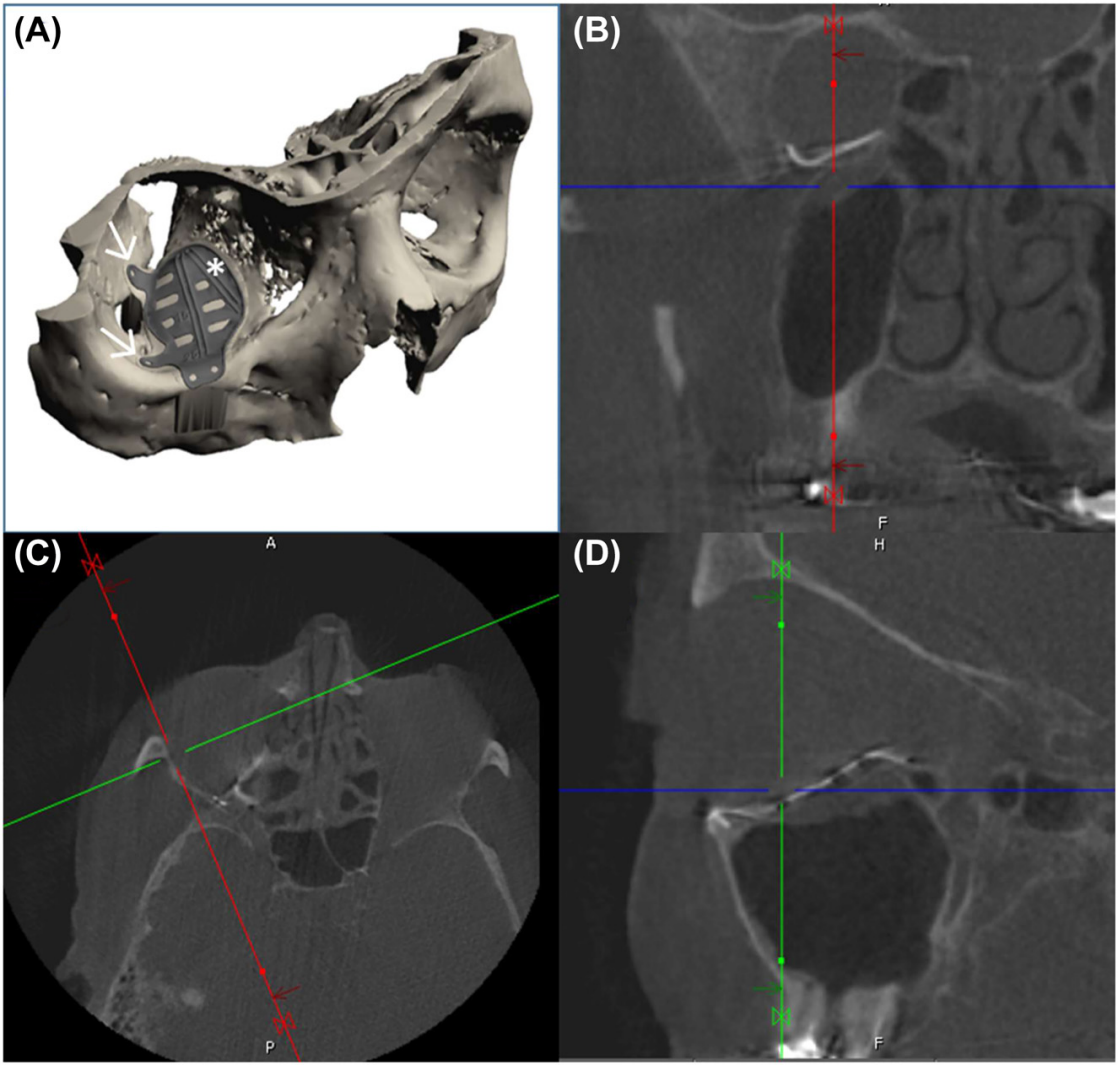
Virtual planning of orbital reconstruction (A) with a patient-specific and functionalized implant (arrows, extensions for positioning, asterisk, a trajectory) and intraoperative cone beam computed tomography after implant placement for orbital reconstruction (B–D).
